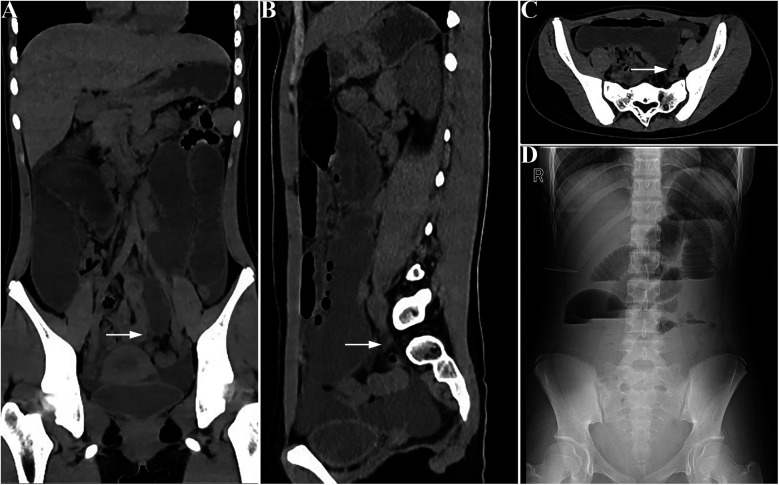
Preoperative presentation: CT imaging reveals significant distension and fluid accumulation in the small intestine, with the distal colon appearing collapsed. (A) coronal view, (B) sagittal view, (C) cross-sectional view; the white arrow indicates the obstruction point). Panel (D) shows an abdominal upright x-ray following preoperative conservative treatment, demonstrating dilated small bowel loops.
